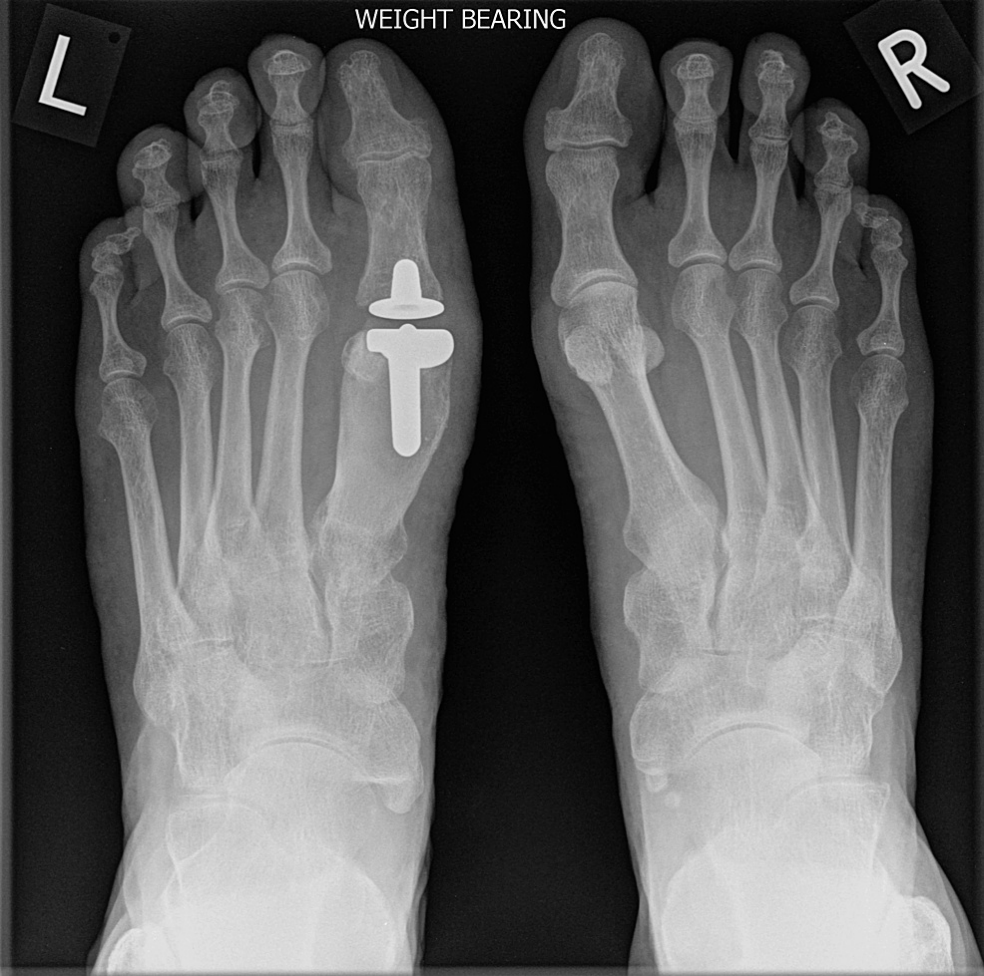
Anteroposterior post-operative X-ray at 36 months following first metatarsophalangeal joint replacement.
